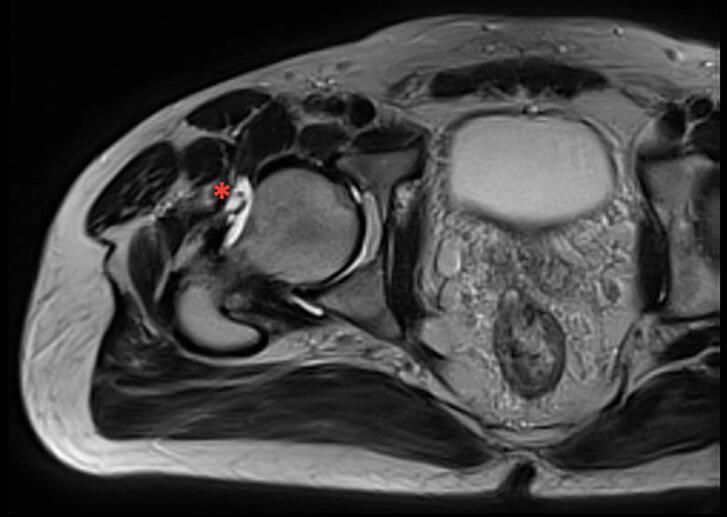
MRI of the right hip showing disruption of anterior capsular ligaments with T2 hyperintensity at the anterior aspect of the femoral head (asterisk), no additional labral tears or other abnormalities were found.
